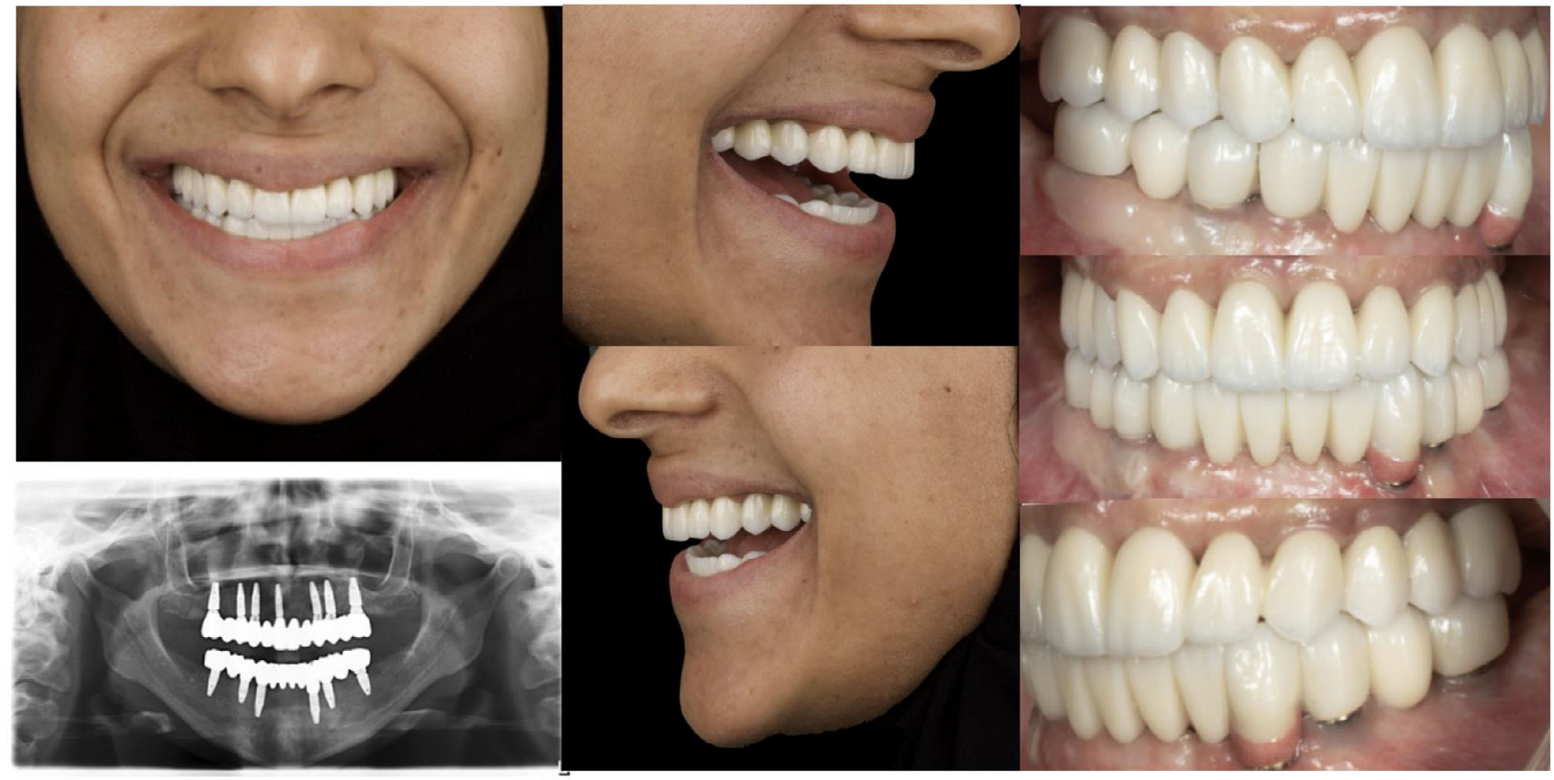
Postoperative extraoral photographs: frontal view, right profile, left profile; postoperative orthopantomogram; and intraoral photographs after delivery of the final milled monolithic zirconia prosthesis.
